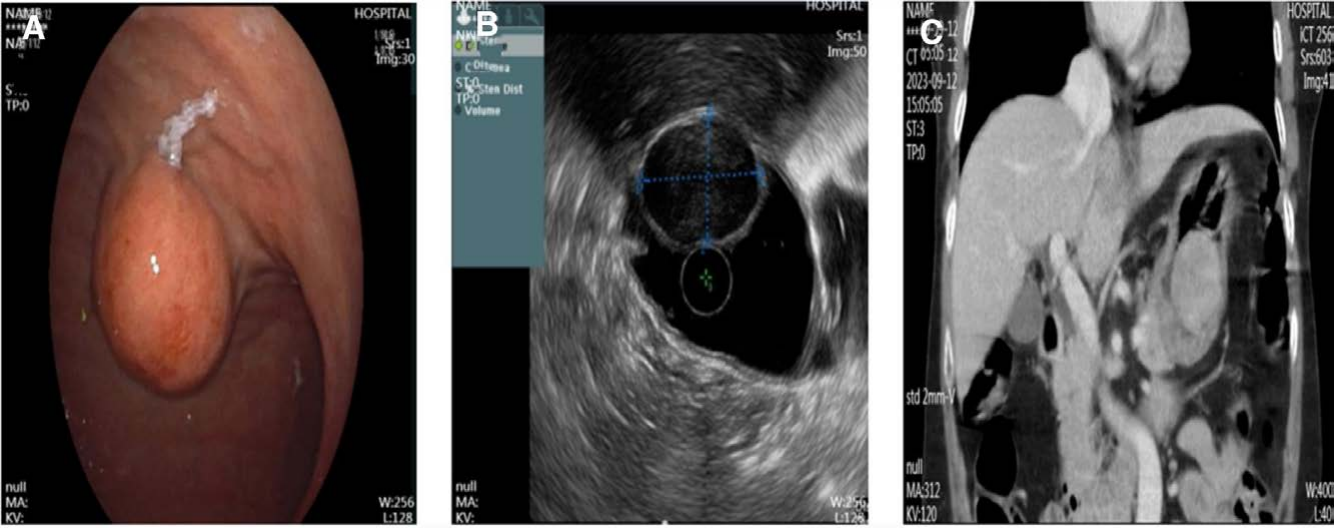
Abdominal computed tomography and endoscopic ultrasonography. (A) White-light endoscopy shows a submucosal bulge on the anterior wall of the lower stomach near the greater curvature. (B) The lesion originates from the muscularis propria and appears as an uneven hypoechoic mass that is primarily intraluminal with no detectable blood flow signals on ultrasonography. (C) Computed tomography scan reveals a quasi-circular mass of slightly lower density on the anterior wall of the stomach with clear boundaries, uniform density, and uniform enhancement with contrast.
